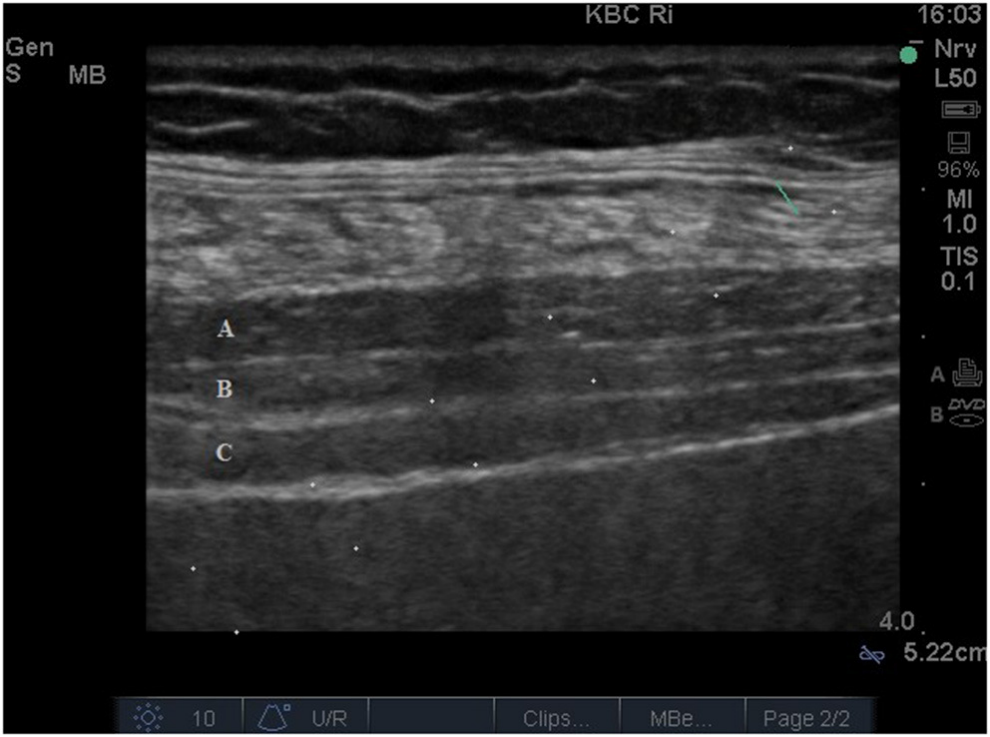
Ultrasound image showing all three muscles of the abdominal wall: (A) external oblique, (B) internal oblique and (C) transversus abdominis muscle.
